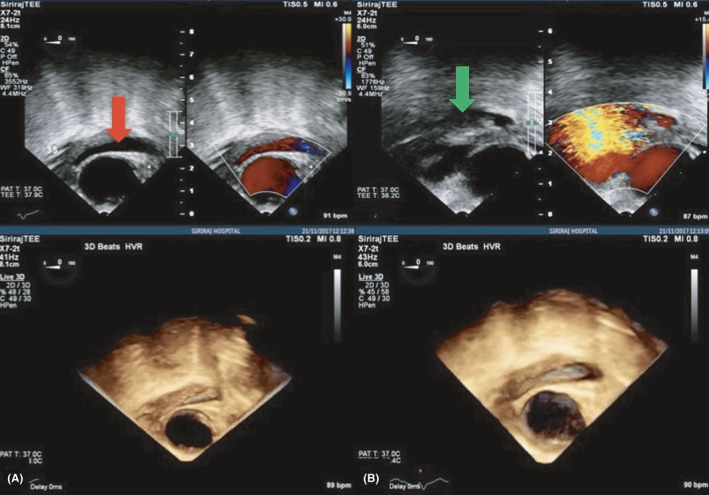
Intraoperative transesophageal echocardiogram after TAVR showing the further extent of the echo‐lucent area in the descending aorta by serial TEE from A (Red arrow) to B (Green arrow)
